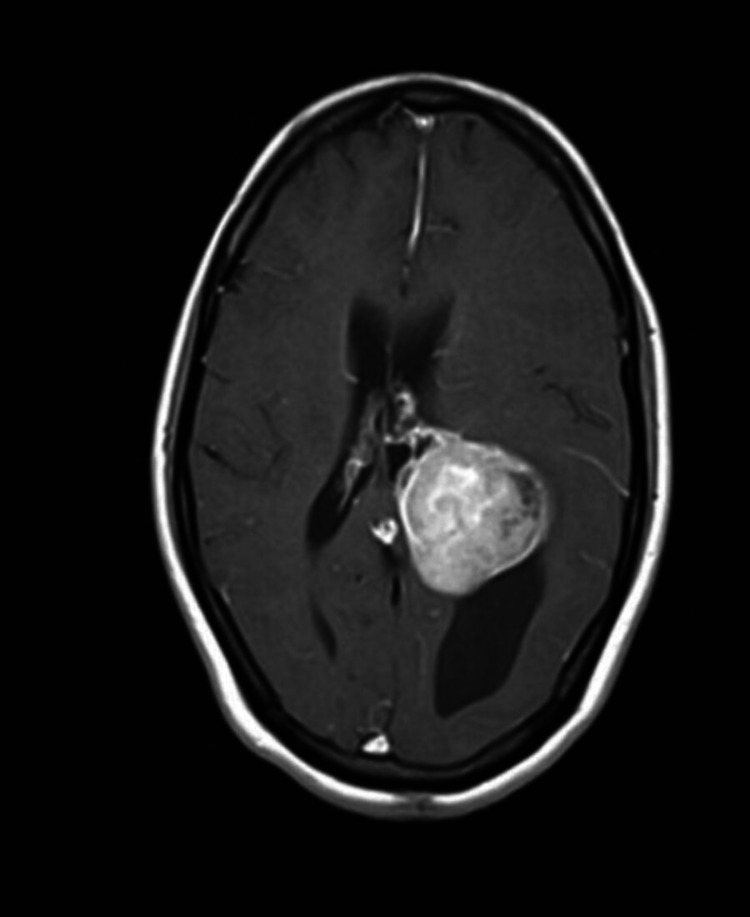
Preoperative MRI brain contrast - B
